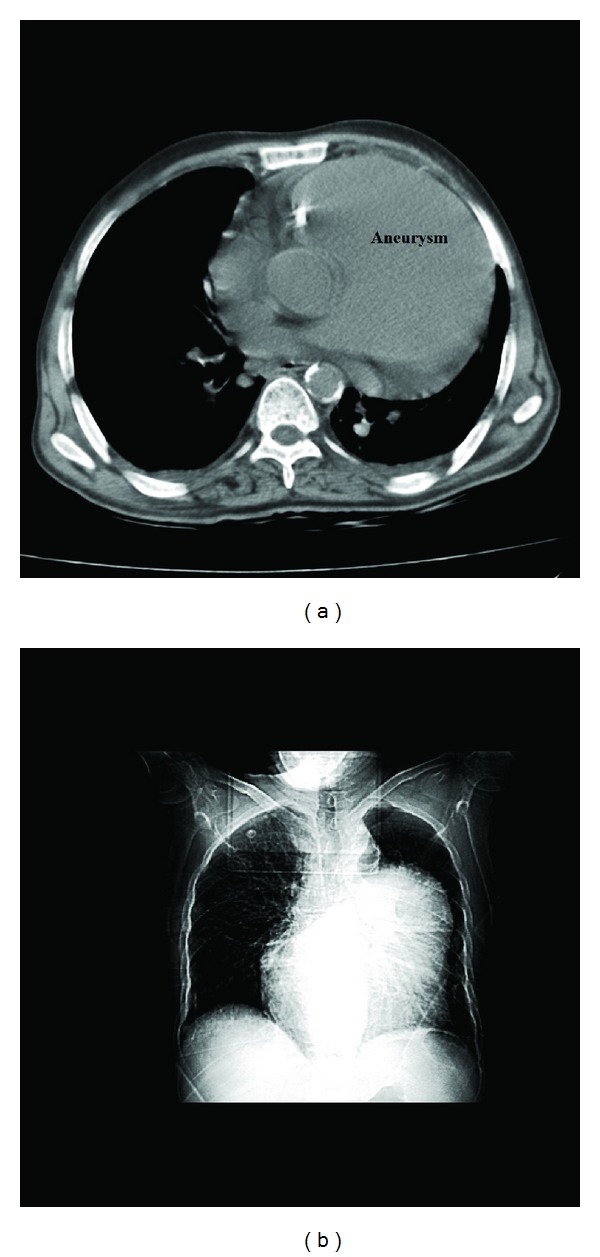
Patient's computed tomography and chest radiography (b) confirmed diagnosis.
